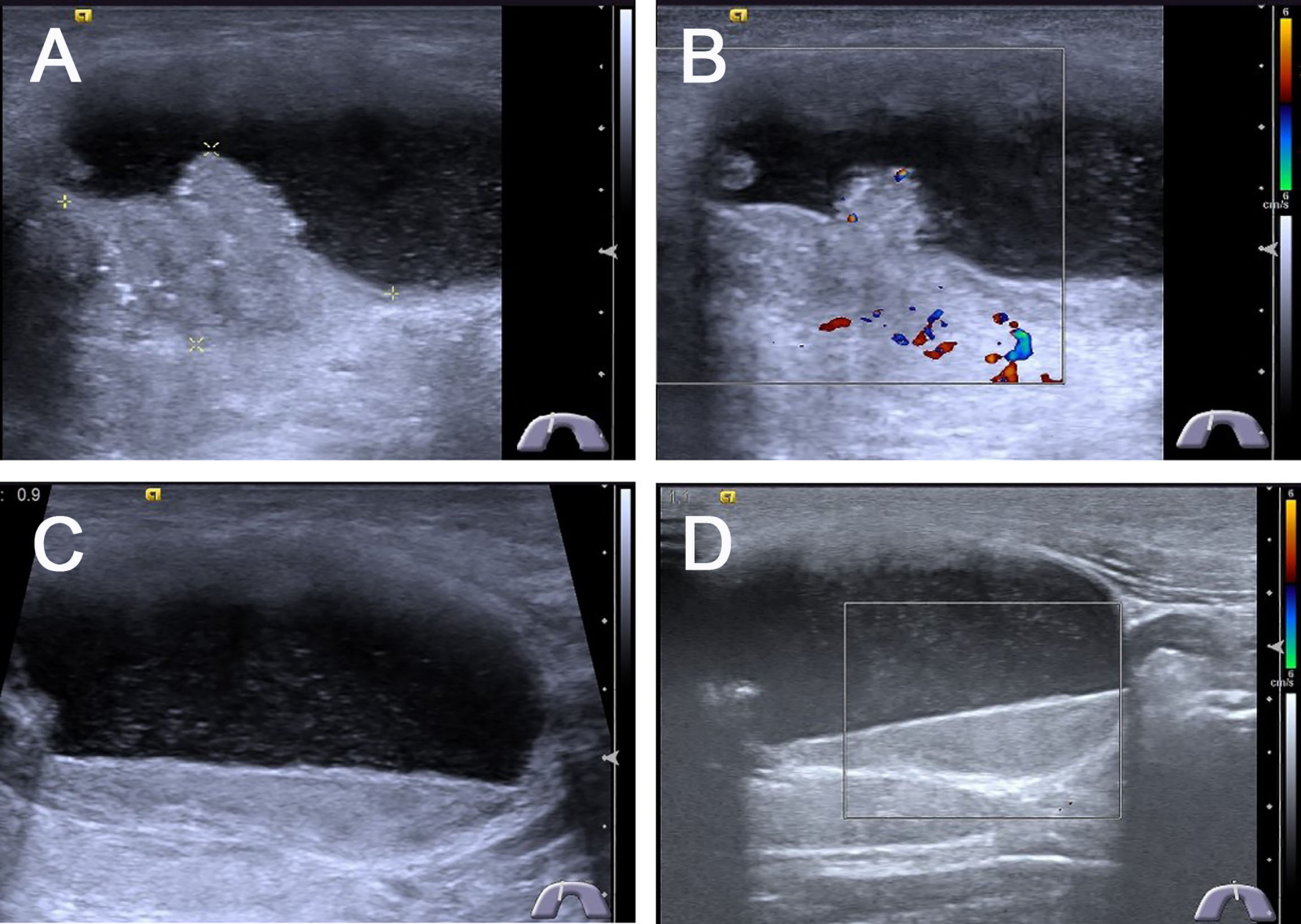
Conventional ultrasonography images of predominantly cystic papillary thyroid carcinoma. (A) Longitudinal gray-scale sonography revealed a predominantly cystic 4.2 × 2.4 × 2.7 cm^3^ thyroid nodule with a little solid portion abutted on the side of the cyst wall in the thyroid isthmus, the cystic portion was more than 90% of the thyroid node. (B) CDFI showed poor blood flow signals in the solid portion of the thyroid nodule. (C) Most portions of this thyroid node were cystic with a lot of mobile silt-like isoechoic substance. (D) CDFI showed no blood flow signals in the silt-like isoechoic substance.
